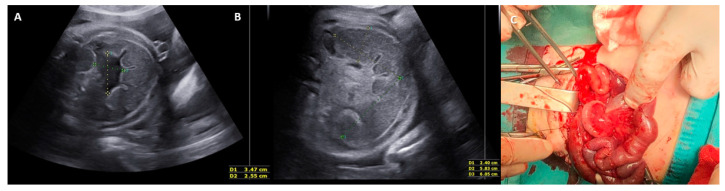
Case of fetal intestinal volvulus. (A,B) Fetal ultrasonography at 34 weeks of gestation: snail-like distended bowel loop with ascites; (C) intraoperative findings of the ileal volvulus with necrosis and distal atresia.
